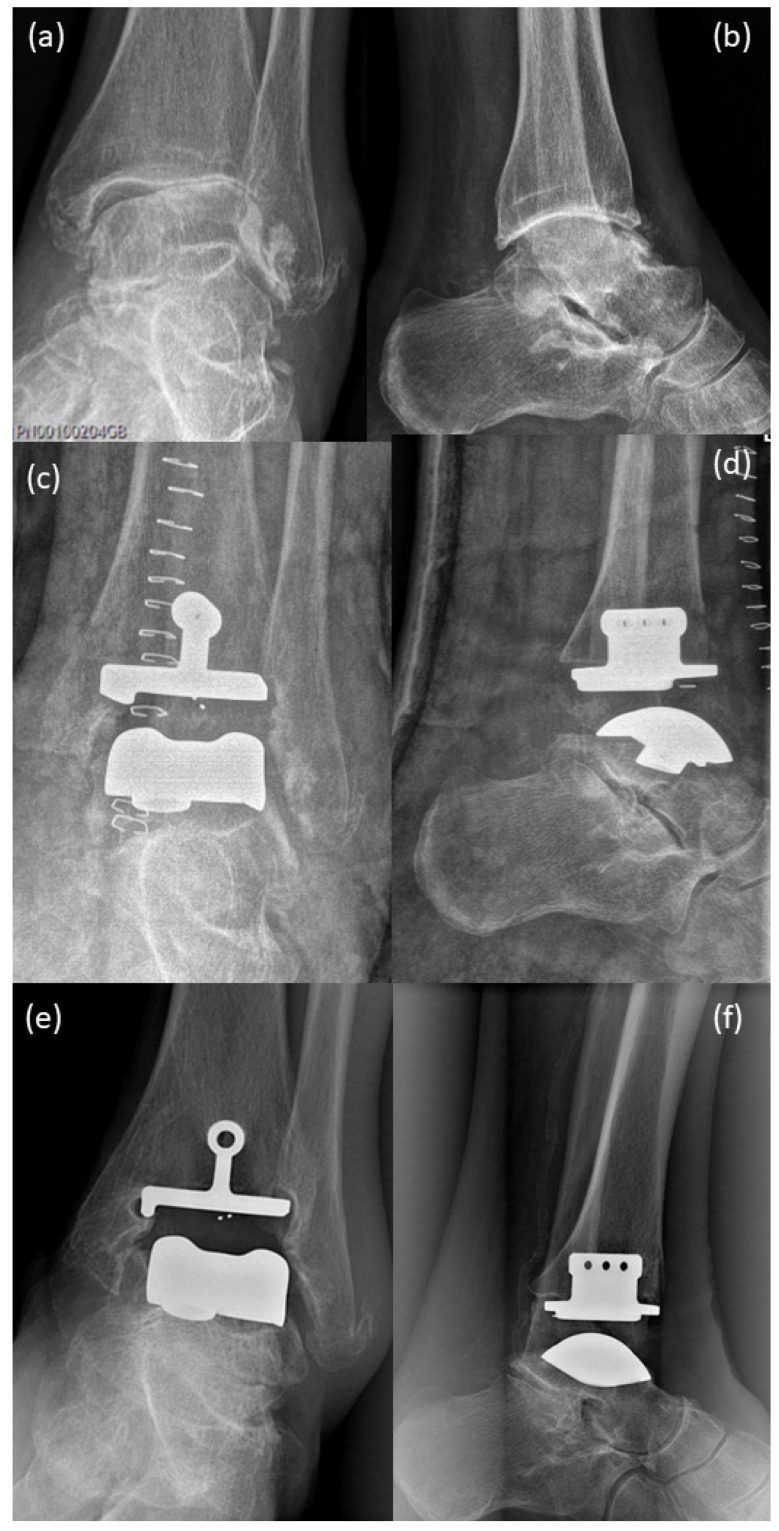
X-rays: (a,b) before implantation of Salto-prosthesis, pre-OP 2005; X-rays show severe osteoarthrosis of the upper ankle with deformity of joint endplates, osteophyte formation and subchondral sclerosis, as well as periarticular calcifications; (c,d) post-surgical 2006, implanted Salto-prosthesis; with regular post-surgical status; (e,f) X-ray at a routine control performed in 2016 demonstrated beginning of bone resorption and lytic–cystic defects in the periphery of the tibial component while still regularly around the stem of the prosthesis and the talar component.
